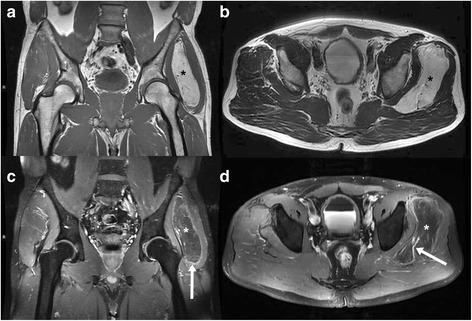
48-year-old man with hibernoma of the left upper leg. Tumour between the gluteus medius and minimus muscles (star in image 1 a-d). MRI shows an isointense tumour compared to fat with slight rim enhancement (arrow in c) and a prominent vessel within the mass (arrow in d). a plain T1-weighted-TSE (TR 819 ms/ TE 11 ms), b plain T2-weighted-TSE (TR 5050 ms/ TE 96 ms), c contrast enhanced fat saturated T1-weighted-TSE (TR 895 ms/ TE 11 ms), d contrast enhanced fat saturated T1-weighted-TSE (TR 680 ms/ TE 11 ms).
